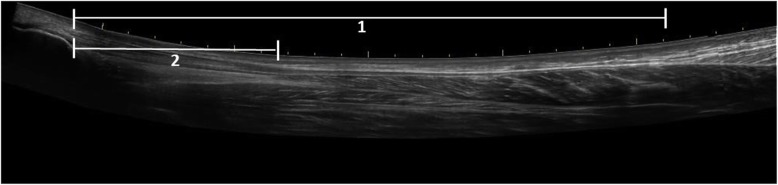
Copenhagen Achilles Length Measure (CALM): an ultrasonographic measurement of tendon elongation. CALM comprises two measurements: the total length of the Achilles tendon (distance 1) and the free length of the Achilles tendon (distance 2)
